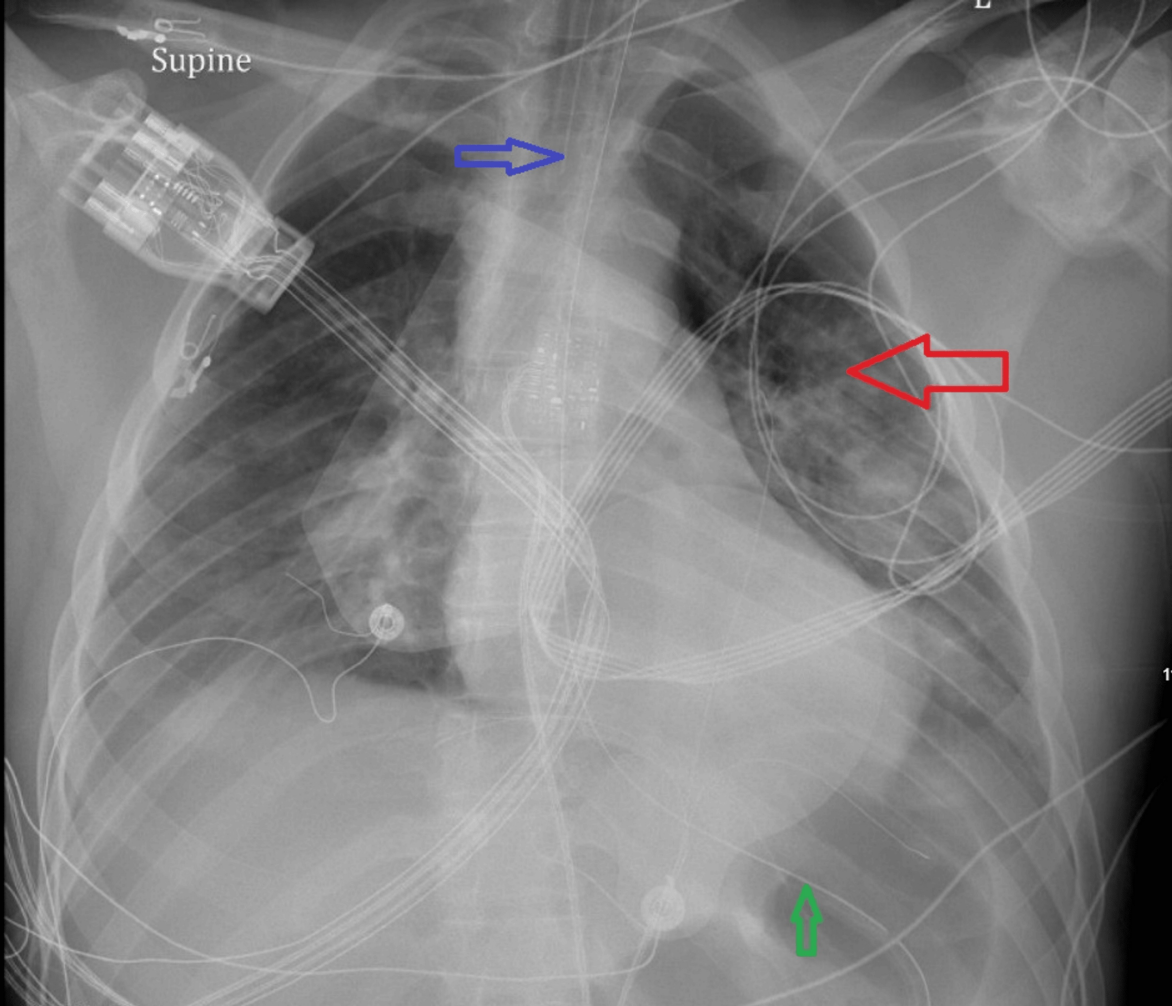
Chest X-ray obtained after endotracheal tube intubation. The red arrow shows prominent infiltrates on the left side. The blue arrow shows the endotracheal tube. The green arrow shows the nasogastric tube.
